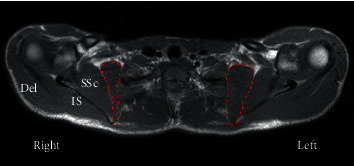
T*1-weighted magnetic resonance image showing slight atrophy of the serratus anterior muscle (dotted line) on the right side compared with left side in the axial plane. SSc: subscapularis muscle; IS: infraspinatus muscle; Del: Deltoid muscle.
